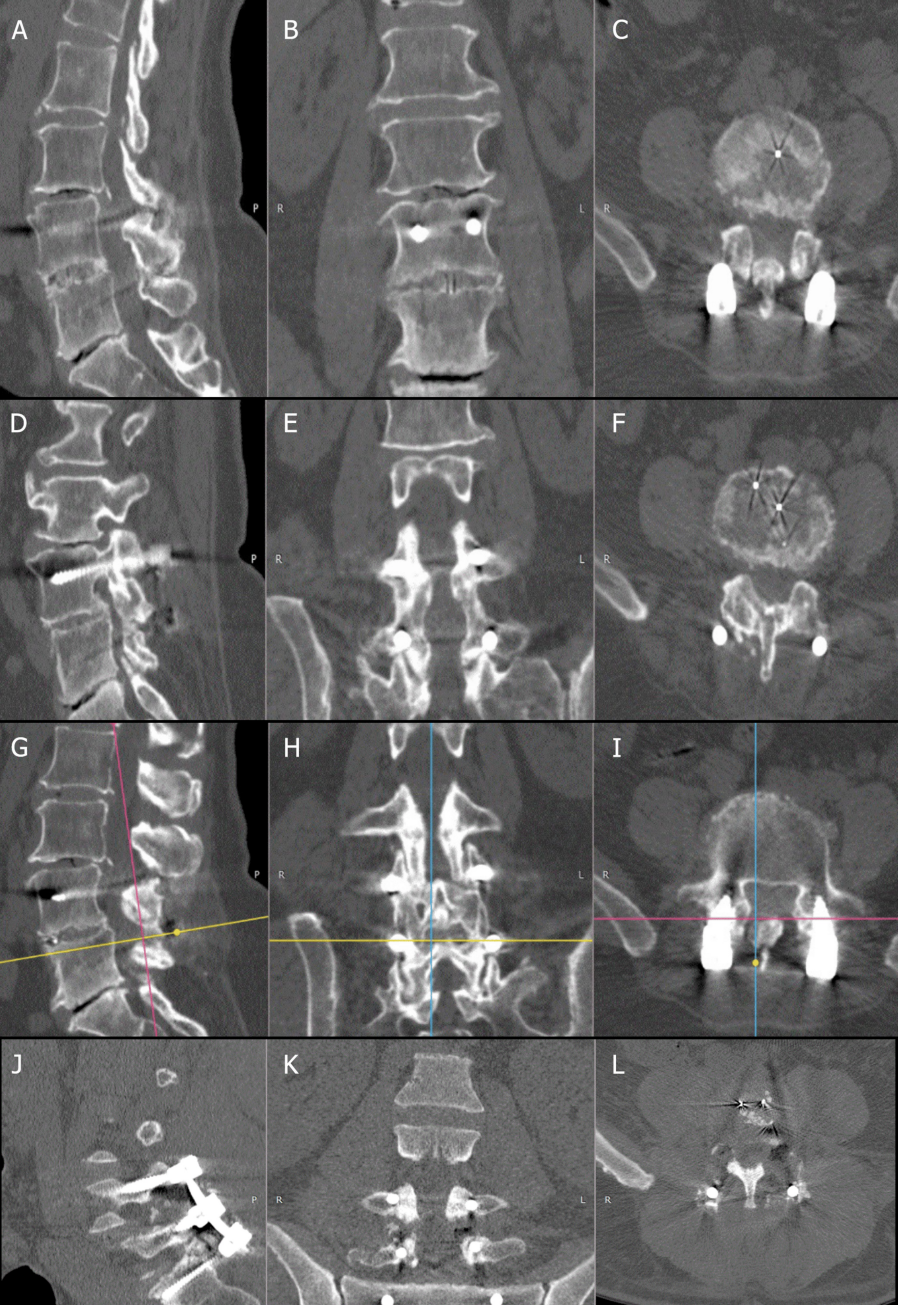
Multiplanar CT adjudication of lumbar fusion across four anatomical sites from the study cohort. Examples shown on bone-window reformats (slice thickness ≤1.25 mm; bone kernel) in the three orthogonal planes. A–C, Intertransverse (posterolateral) space: sagittal (A), coronal (B), axial (C); fusion = trabecular bridging across the decorticated intertransverse gutters and adjacent transverse processes. D–F, Interbody space: sagittal (D), coronal (E), axial (F); fusion = continuous trabecular bone traversing the adjacent endplates through/around the cage without an intervening cleft. G–I, Facet joint space: sagittal (G), coronal (H), axial (I); fusion = osseous bridging/obliteration of the facet joint after decortication. J–L, Posteromedial space: sagittal (J), coronal (K), axial (L); fusion = bridging along the lamina/spinous processes within the medial gutters. Operational rule: fusion required continuous trabecular bridging on ≥2 consecutive slices in the same plane or confirmation on orthogonal planes within the same zone.
